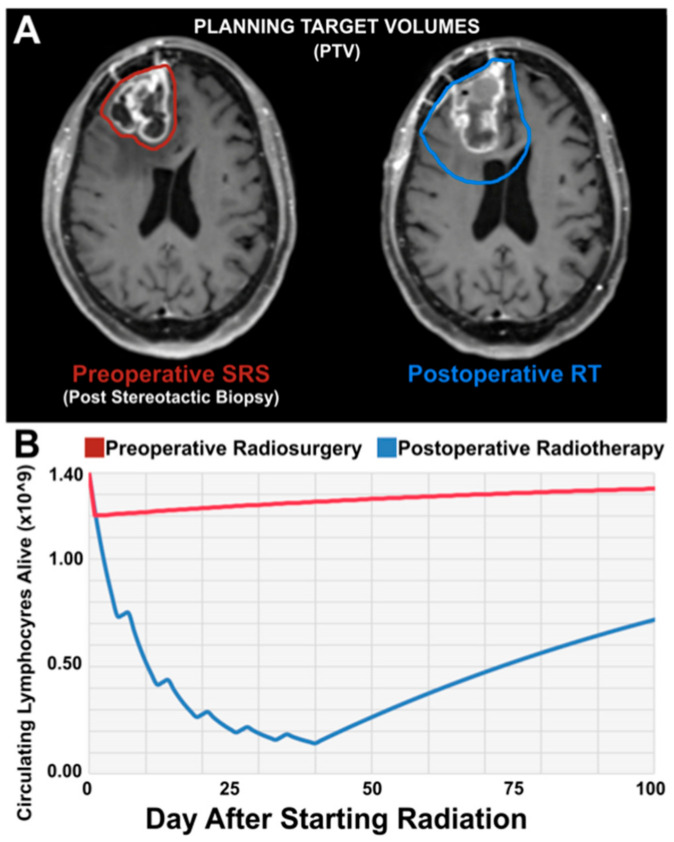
Preoperative SRS irradiates less healthy brain and kills less lymphocytes compared to Postoperative RT. (A) Preoperative (left) and postoperative (right) MRI showing preoperative SRS PTV (red) as compared to standard postoperative RT PTV (blue). As demonstrated, the vast majority of tissue irradiated during preoperative SRS is resected and minimal dose of radiation is delivered by SRS beyond the tumor bulk. (B) Changes in serum naive CD8+ lymphocytes after SRS compared to postoperative RT based on our preliminary mathematical modeling. Staggered edge reflects weekend recovery.
